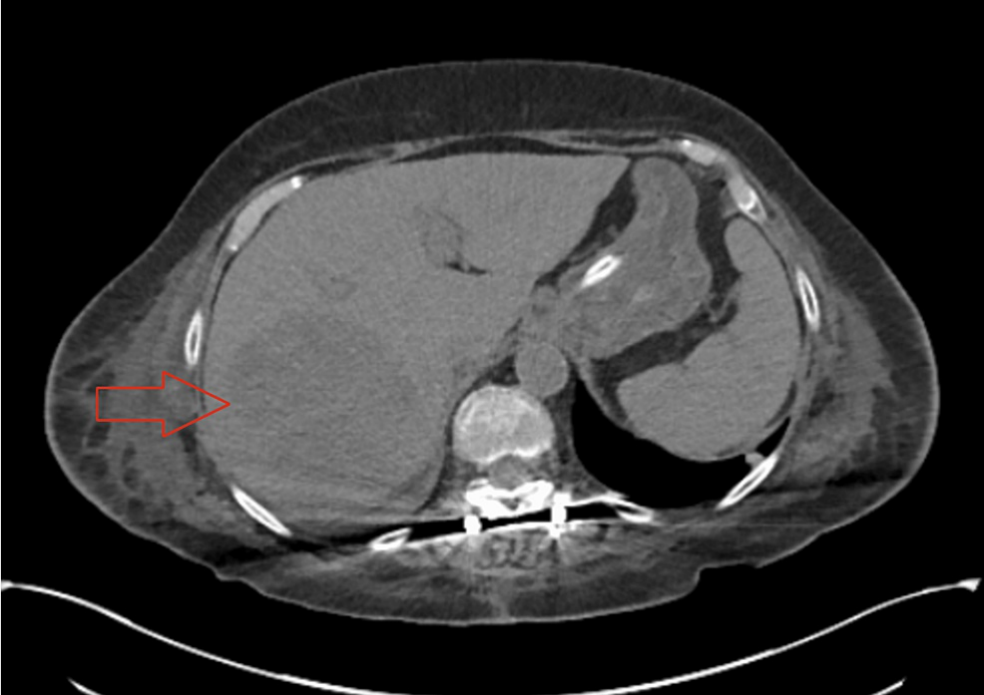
Multiple liver lesions are present on the CT Abdomen of the axial section. The largest occupies a significant portion of the right lobe of the liver and if it does not represent two lesions adjacent to one another measures overall close to 9.4 cm. The contour of the liver is mildly irregular.
